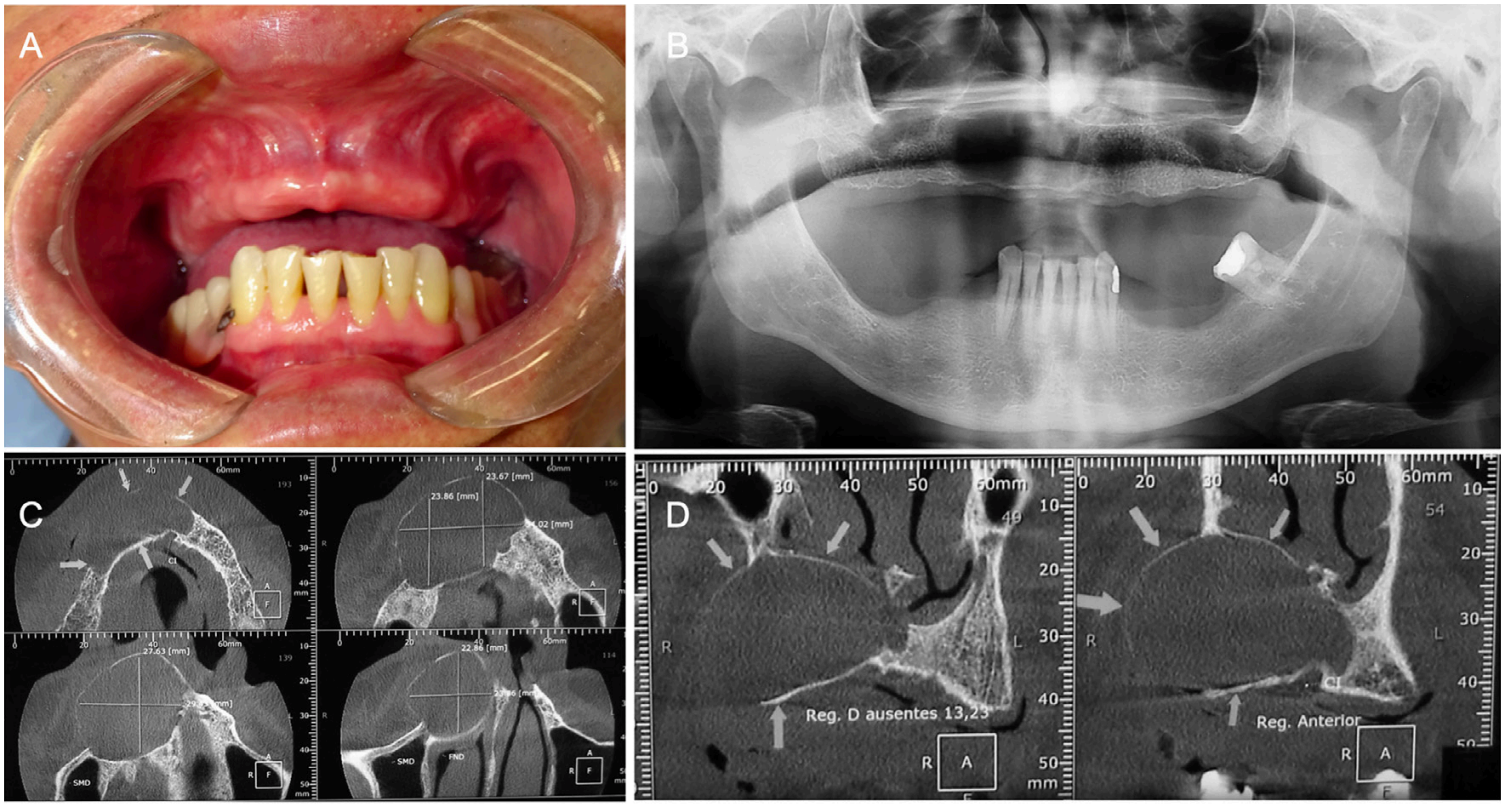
Case 1 clinical and radiographic features. A, Intraoral examination showed a maxillary swelling covered by normal oral mucosa. B, A well-defined unilocular radiolucent image could be observed on panoramic radiograph, mimicking a residual cyst. C-D, CBCT features demonstrating a hypodense lesion causing maxillary cortical expansion without perforation.
